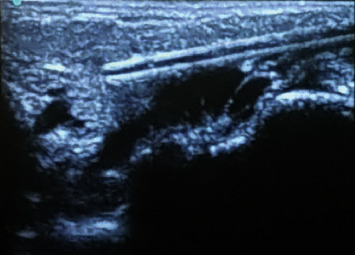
Cannulation of the cystic lesions using ultrasound guidance.
